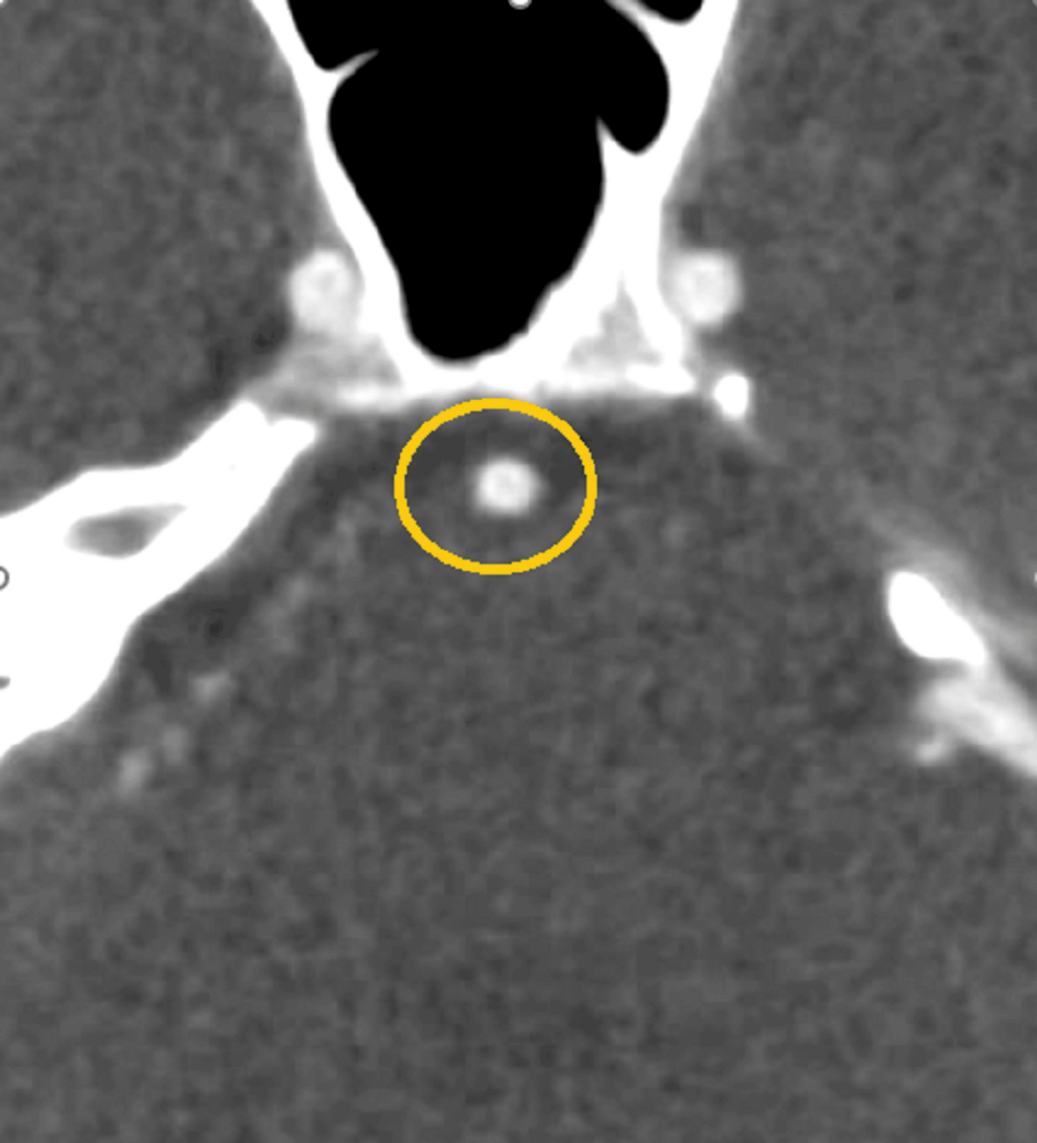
CT angiogram, axial image at the level of the mid-basilar artery, retrospectively showing a possible central filling defect (donut sign).
